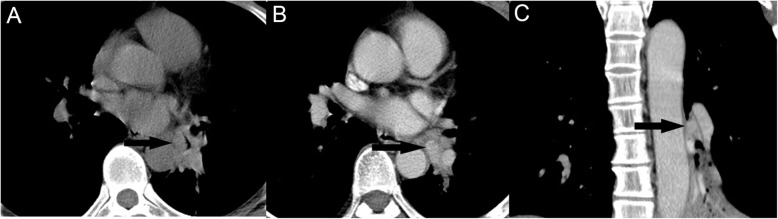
A PMEC with regular shape and well-defined margin with hilar type. Axial non-contrast CT image (a) shows a isodense mass (arrows) in left inferior lobar bronchus. Contrast-enhanced CT image (b, c) shows tumor with marked enhancement without necrosis (arrows)
